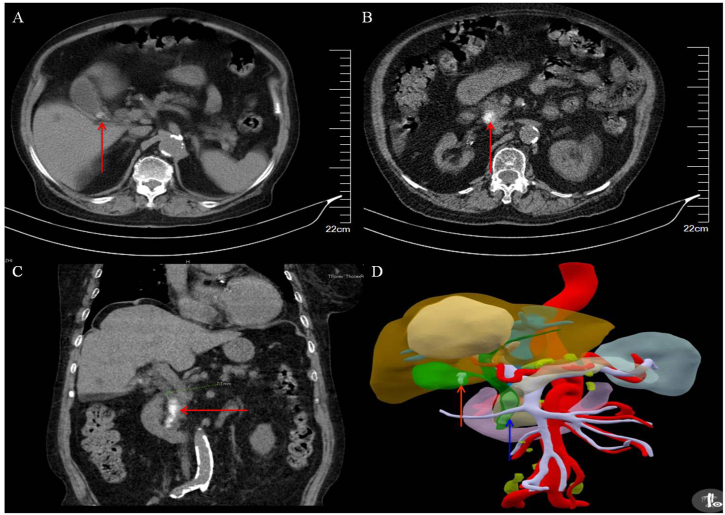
Preoperative CT in a 96-year-old female patient with ACC + CBDS. A: Preoperative axial CT image showed a circular (diameter 8 mm, arrow) high-density shadow at the neck of gallbladder, with a bilateral sign on the gallbladder wall; B: a circular (diameter 15 mm, arrow) high-density shadow within the CBD; C: Coronal CT image displayed multiple nodular high-density shadows in the CBD; D: CT three-dimensional (3D) visualization reconstruction image confirmed the smaller high-density shadow at the neck of the gallbladder (red arrow), and the larger high-density shadows in the CBD (blue arrow). ACC: Acute calculous cholecystitis. CT: Computed tomography. CBD: Common bile duct. CBDS: Common bile duct stone.
